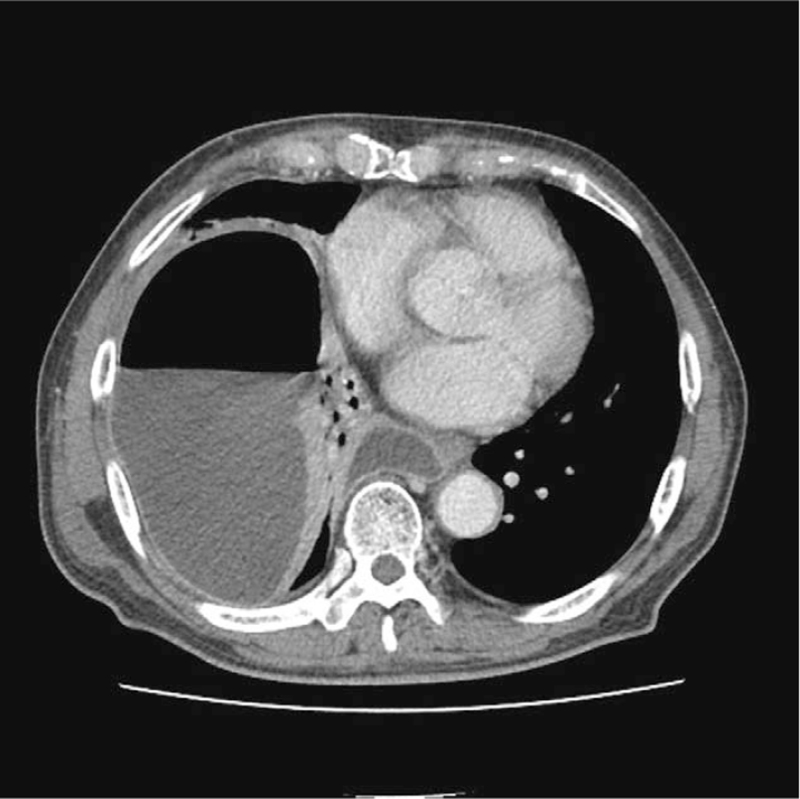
Chest computed tomography findings. Multilobulated pleural effusion and air-fluid level in the right pleural space.
